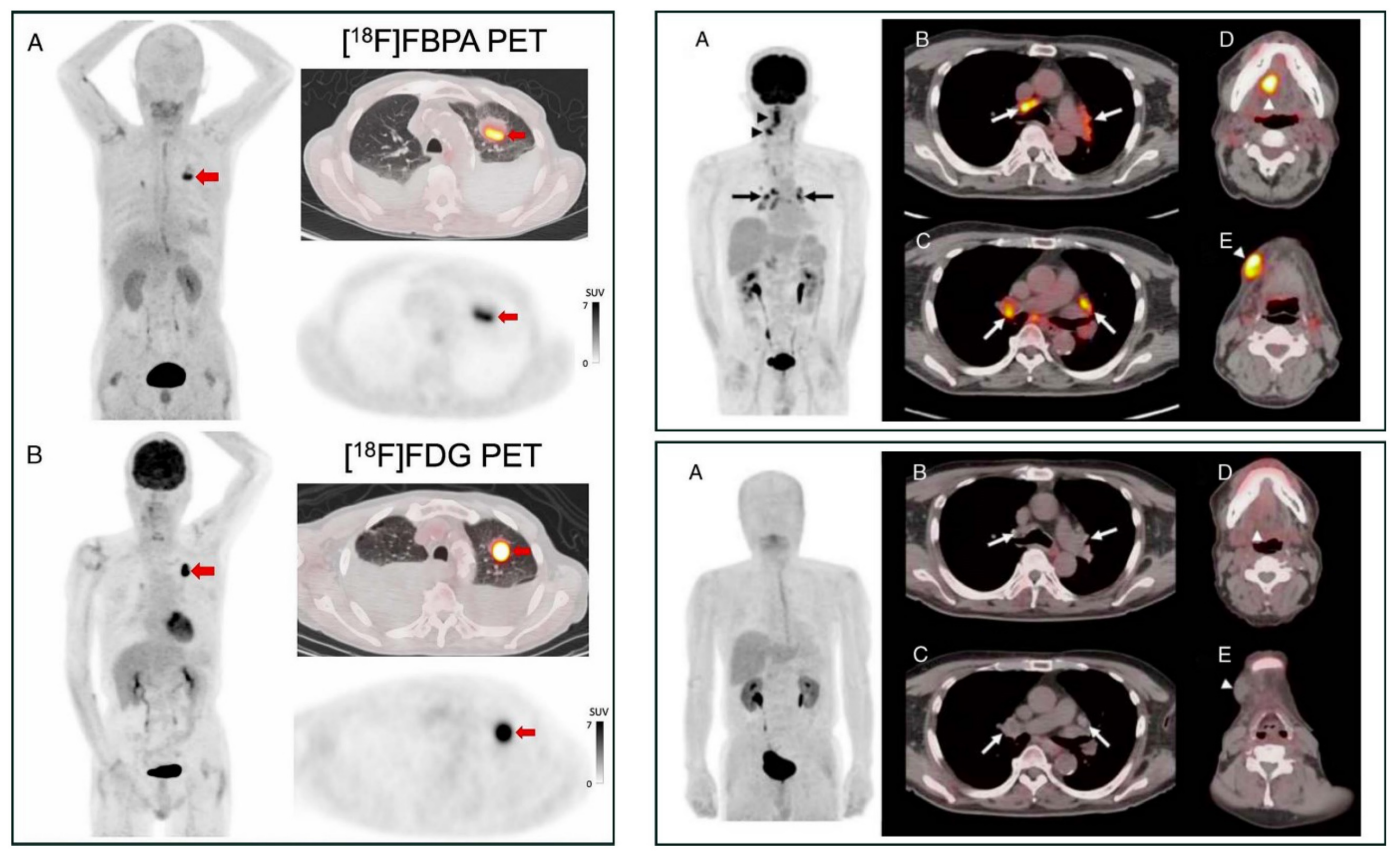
PET MIP and axial PET/CT image comparison between [^18^F]FBPA and [^18^F]FDG. Left box: A primary lung squamous cell carcinoma on a 71-year-old man (*red arrows*, [^18^F]FDG SUV_max_ 14.8 and [^18^F]FBPA SUV_max_ 7.2). Reproduced as is from 66. Copyright © 2023, Copyright © 2023 The Author(s). Published by Wolters Kluwer Health, Inc. Originally published under Creative Commons Attribution-Non Commercial-No Derivatives License 4.0 (CCBY-NC-ND license). Right boxes: A tongue cancer and mediastinal sarcoidosis case in a 68-year-old man. The [^18^F]FDG PET images *(upper box)* and [^18^F]FBPA PET images *(lower box)* were taken ten and eleven days following high-dose brachytherapy for his tongue cancer, respectively. [^18^F]FDG PET images visualized active inflammation in his mediastinal sarcoidosis (*upper box*, A-C, arrows) and post-radiation inflammation (*upper box*, D & E, arrowheads), while no [^18^F]FBPA uptake in the corresponding area of the mediastinum (*lower box*, A-C, arrows), tongue, and submandibular region (*lower box*, D & E, arrowheads). Reproduced as is from 67. Copyright © 2020, Copyright © 2020 The Author(s). Published by Wolters Kluwer Health, Inc. Originally published under Creative Commons Attribution-Non Commercial-No Derivatives License 4.0 (CCBY-NC-ND license).
